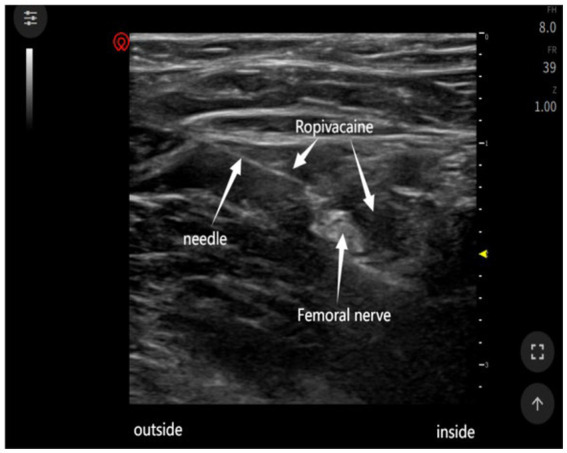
Ultrasound-guided puncture images of the FN.
